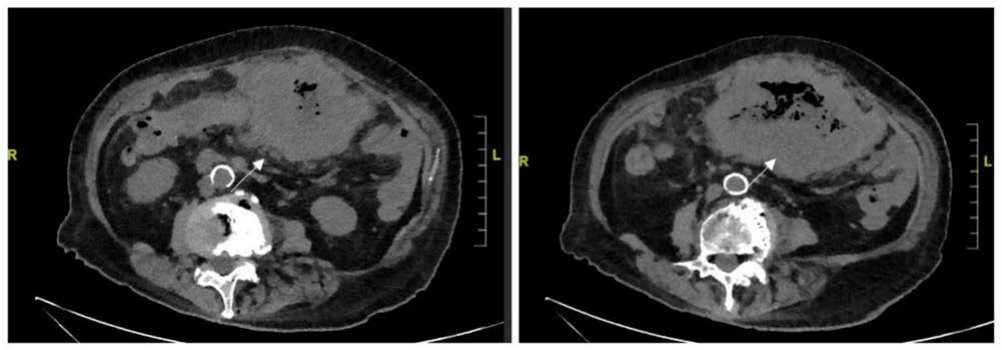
The CT plain scan revealed an irregularly thickened transverse colon wall. The mass was larger than 15 cm (arrows).
